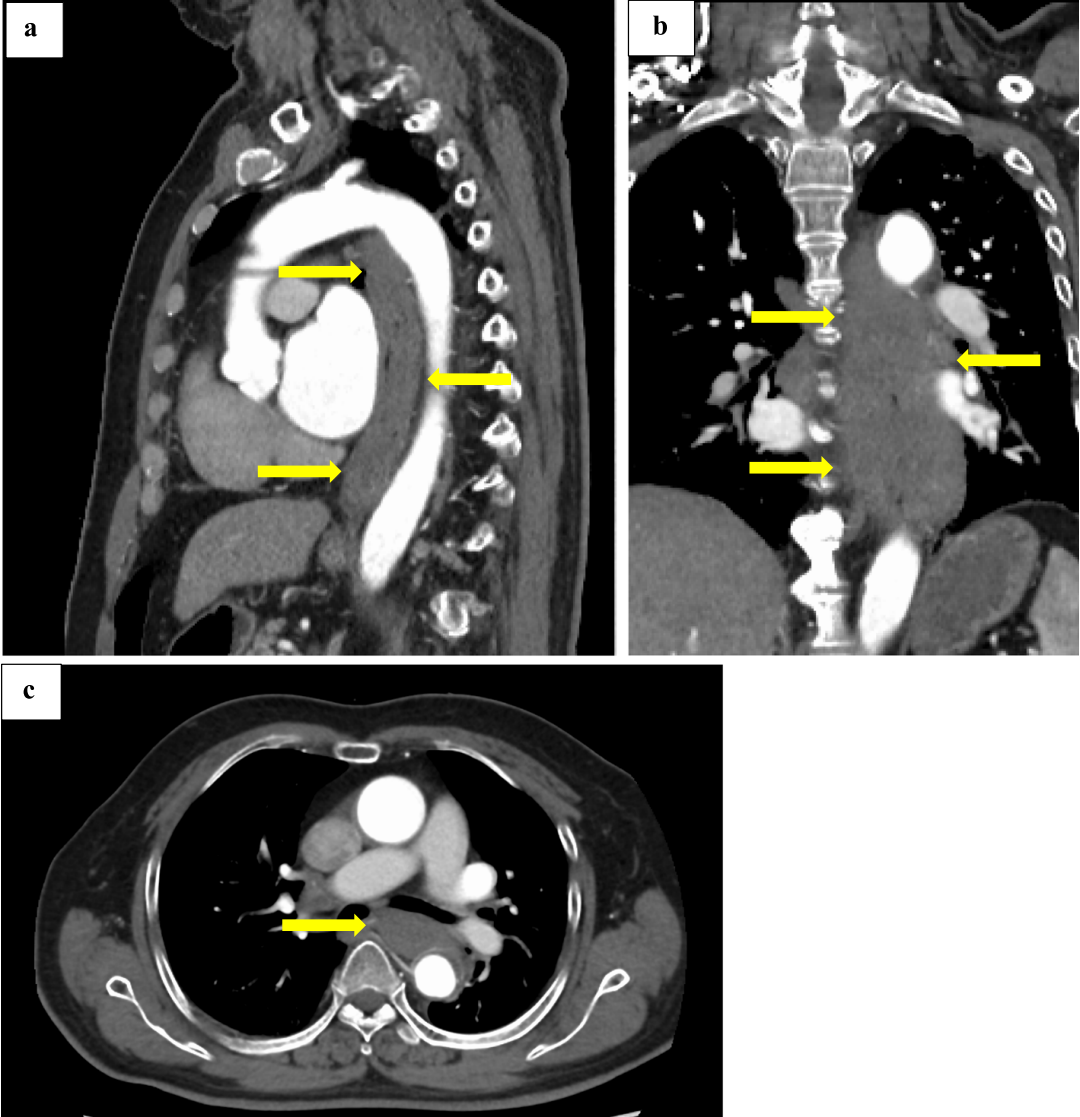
Contrast-enhanced CT at first visit. a Sagittal, b coronal, c axial. Severe edematous wall thickening with unclear contrast effect is evident throughout the esophagus
